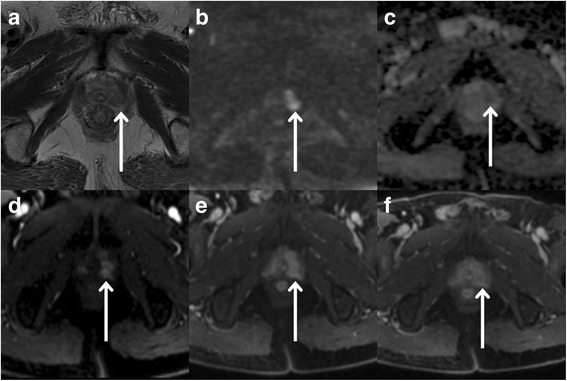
MR images of prostate cancer. a High-resolution T2WI shows low signal intensity area in the left transition (TZ) and peripheral zone (PZ) (arrows). b On ultra-high b-value DWI (b = 3000 s/mm^2^), left-side lesions were observed as significantly higher signal nodules (arrows). c On ultra-high b-value DWI (b = 3000 s/mm^2^) ADC map, ADC value of the left-side lesion was 0.517 ± 0.015 × 10^−3^ mm^2^/s (arrows). d On axial contrast T1WI during the arterial phase, the tumor was enhanced strongly and non-homogeneously (arrows). e, f On axial contrast T1WI during venous and delay phases, the tumor was enhanced strongly and non-homogeneously (continuous signal increase, arrows). The margin of tumor was not clear, and there was no envelope
